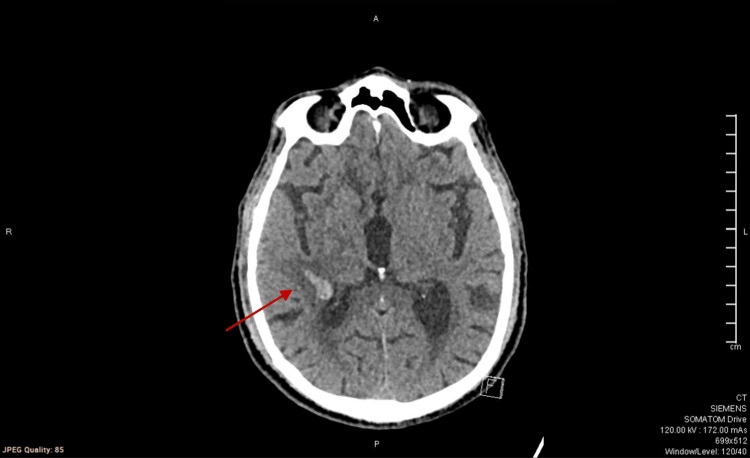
CT of the head without contrast showing a well-defined parenchymal hyperdensity (red arrow) in the right parietal periventricular location, suggestive of parenchymal bleed
